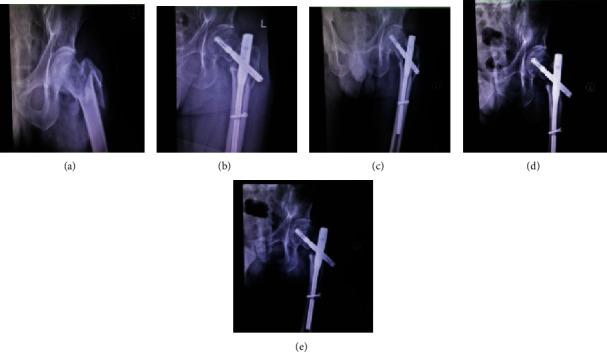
Preoperative and postoperative radiographic data of a patient with reverse oblique intertrochanteric fracture treated with PFNA. Figure notes: (a) preoperative; (b) one month after operation; (c) three months after operation; (d) six months after operation; (e) twelve months after operation.
